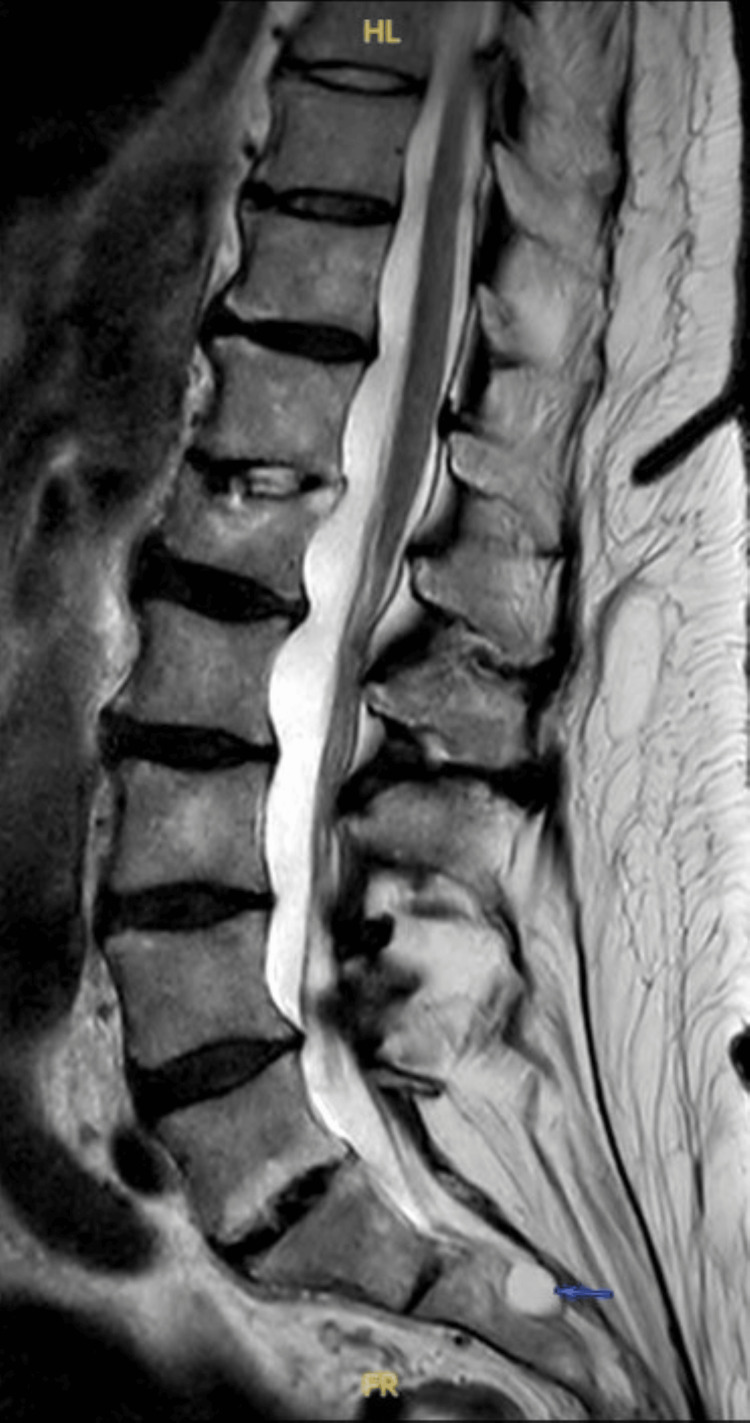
Sagittal T2-weighted MRI showing a sacral Tarlov cyst
